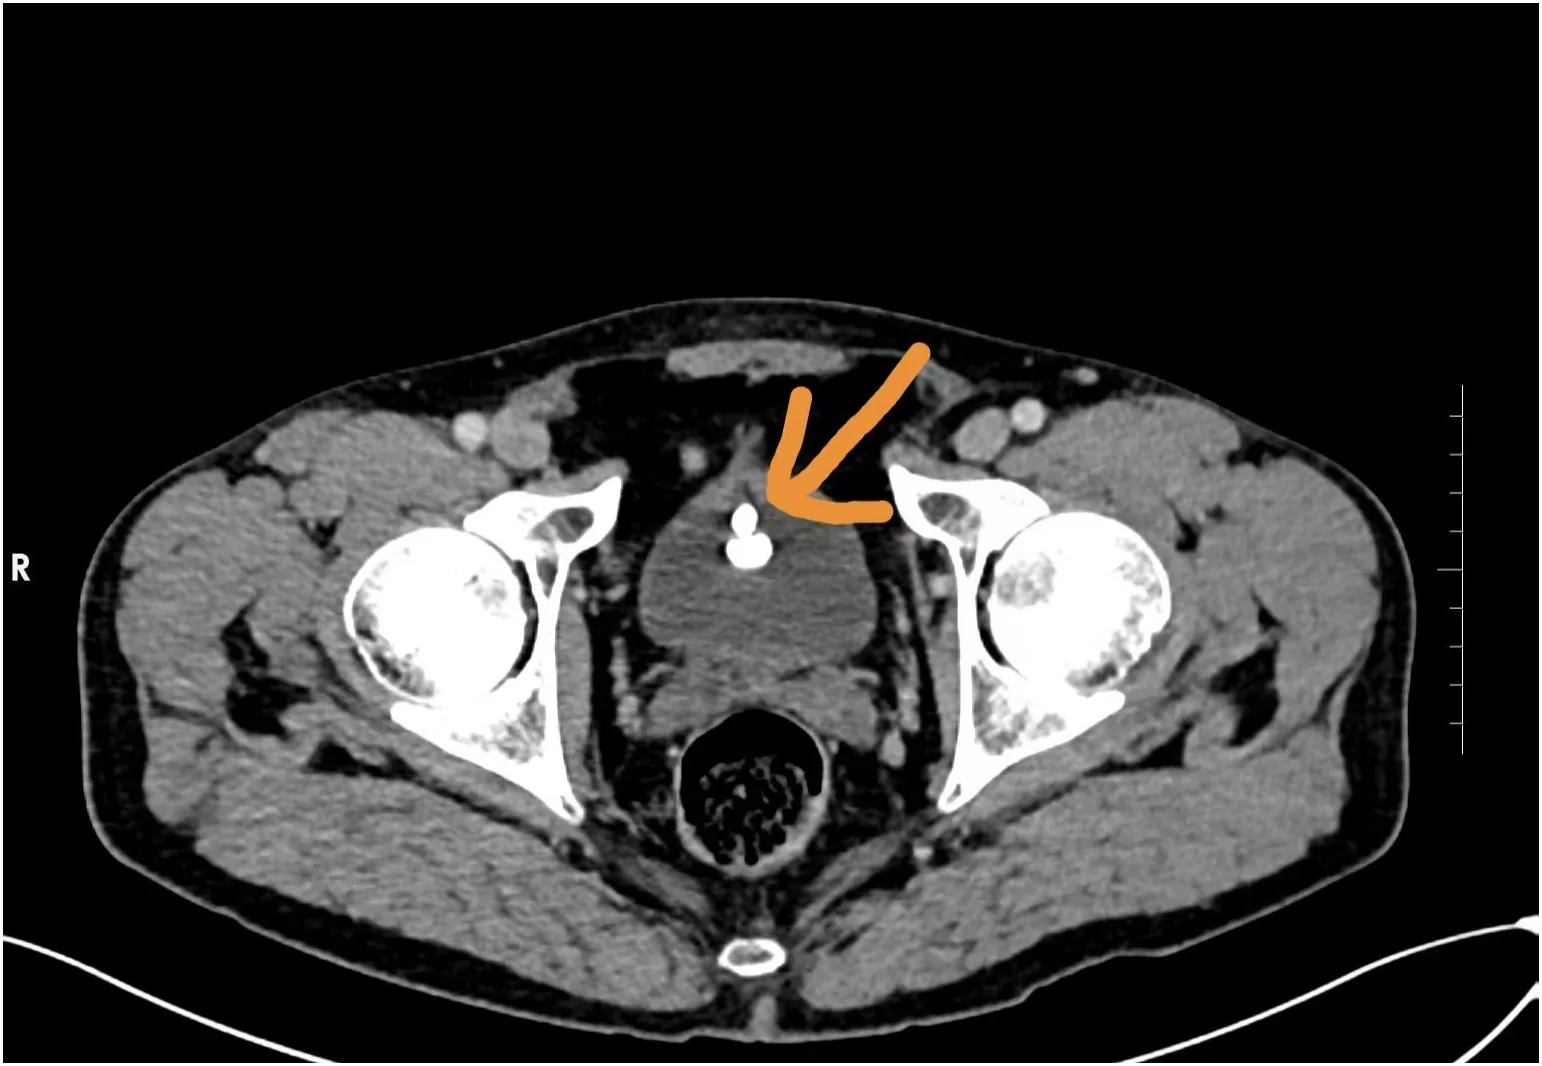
Axial CT image showing a densely calcified nodular lesion at the junction of the bladder dome and anterior wall (arrow), measuring approximately 1.2 x 0.9 cm with an attenuation of approximately 1112 HU.
